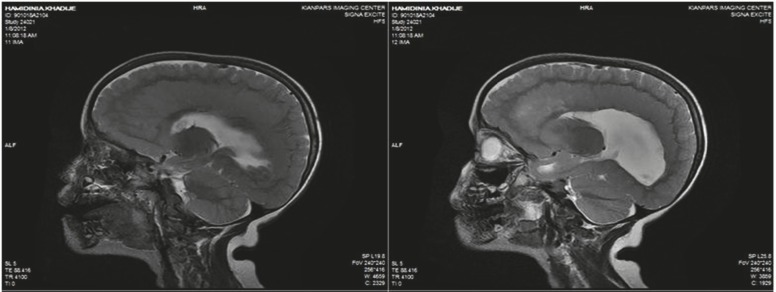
Sagittal T2-weighted brain MRI, shows polymicrogyria, complete band heterotopia
